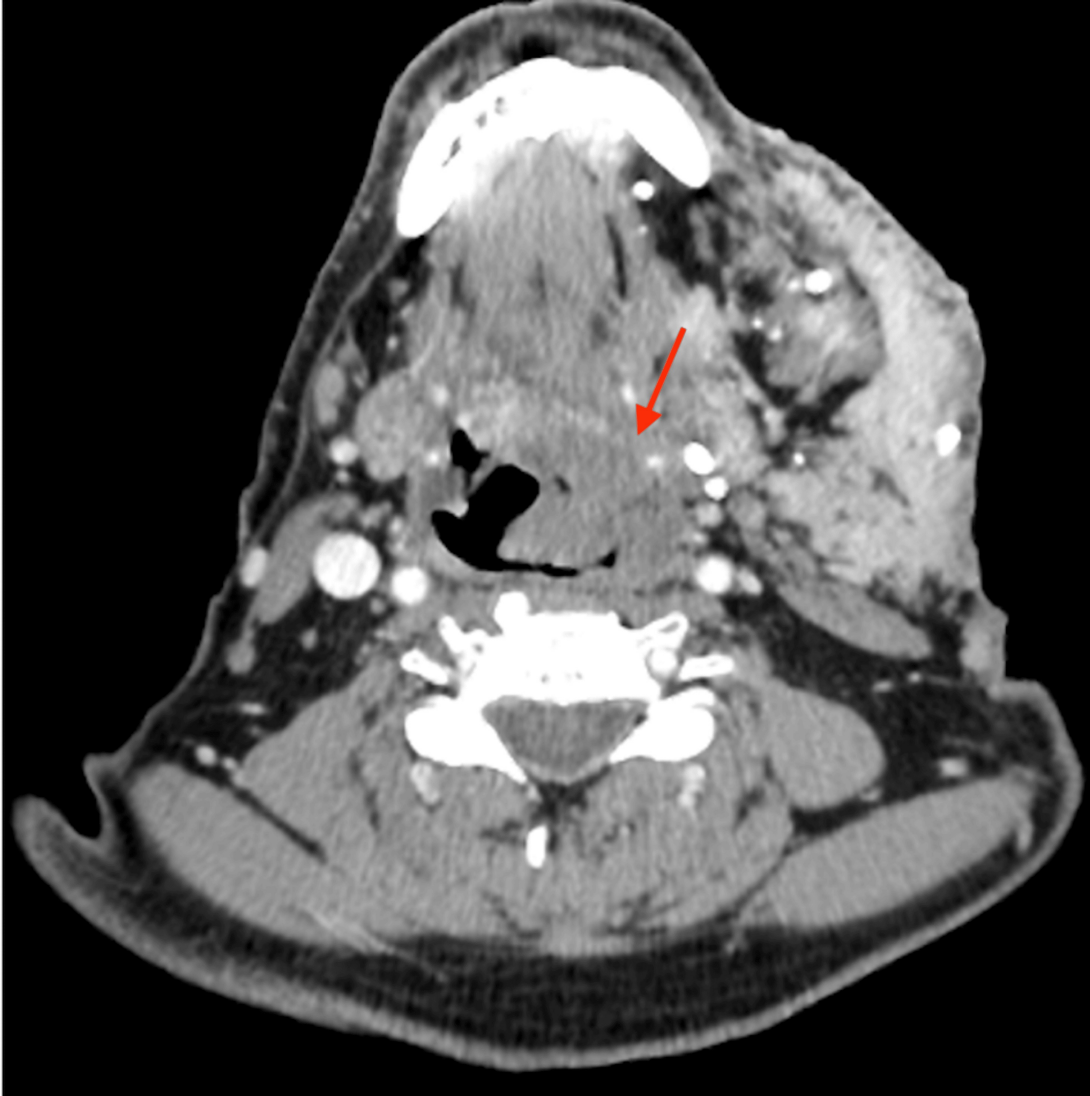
Axial view of a CT scan of the neck at the C3 level of the oropharynx depicting the significant extension of the haemangioma crossing the midline of the airway, as shown by the red arrow CT: Computed tomography
